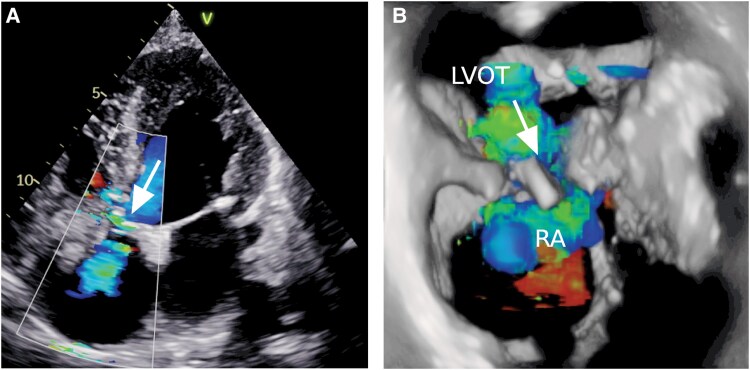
Acquired Gerbode defect (arrow) visualized on transthoracic echocardiography (*A*), transoesophageal echocardiography, and 3D reconstruction (*B*).
